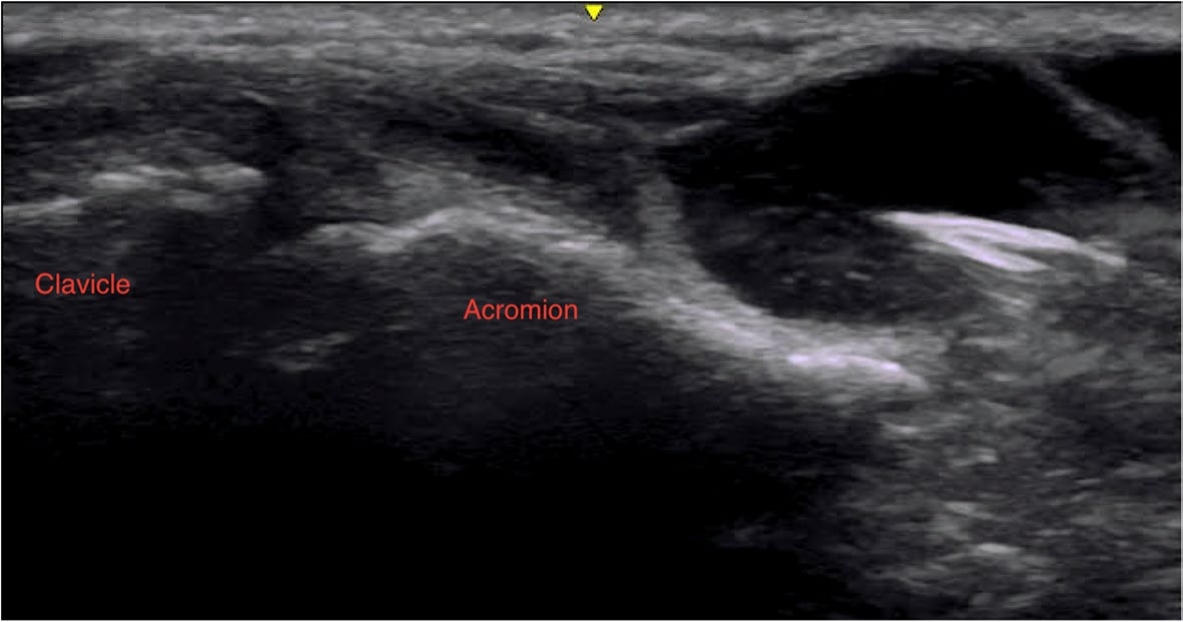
Ultrasound guided aspiration of the AC joint cyst extending from the AC joint capsule
